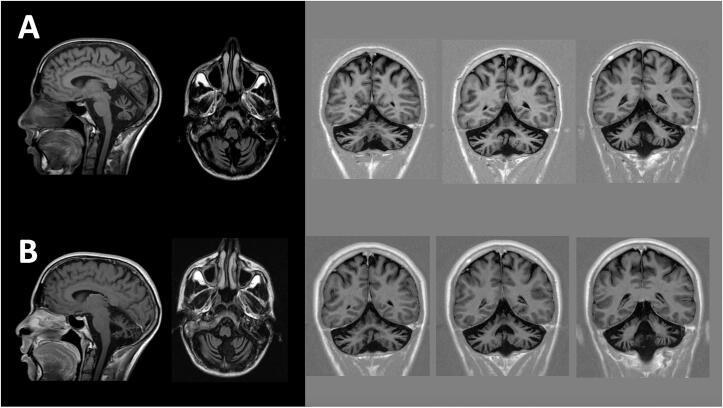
Brain MRI (T1 and T1 inversion-recovery weighted sequences) of the patient was available at baseline (A) and three years later (B), showing marked cerebellar atrophy with preserved brainstem, spinal and supratentorial structures. A slight progression of atrophy can be appreciated in the radiological follow-up, with progression in IV ventricle enlargement. The subject gave consent to be videoed for publication both in print and online.
